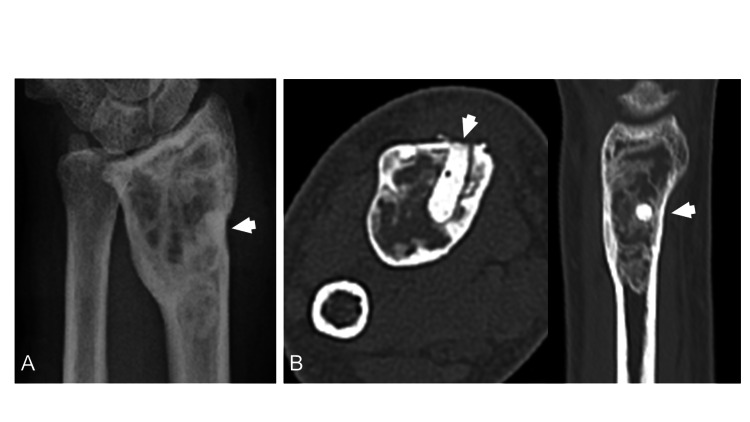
X-rays and CT images taken again before surgery Both X-ray image (A) and CT images (B) showed osteogenesis findings. (Arrows indicated the cement used to fill in the biopsy area.)
